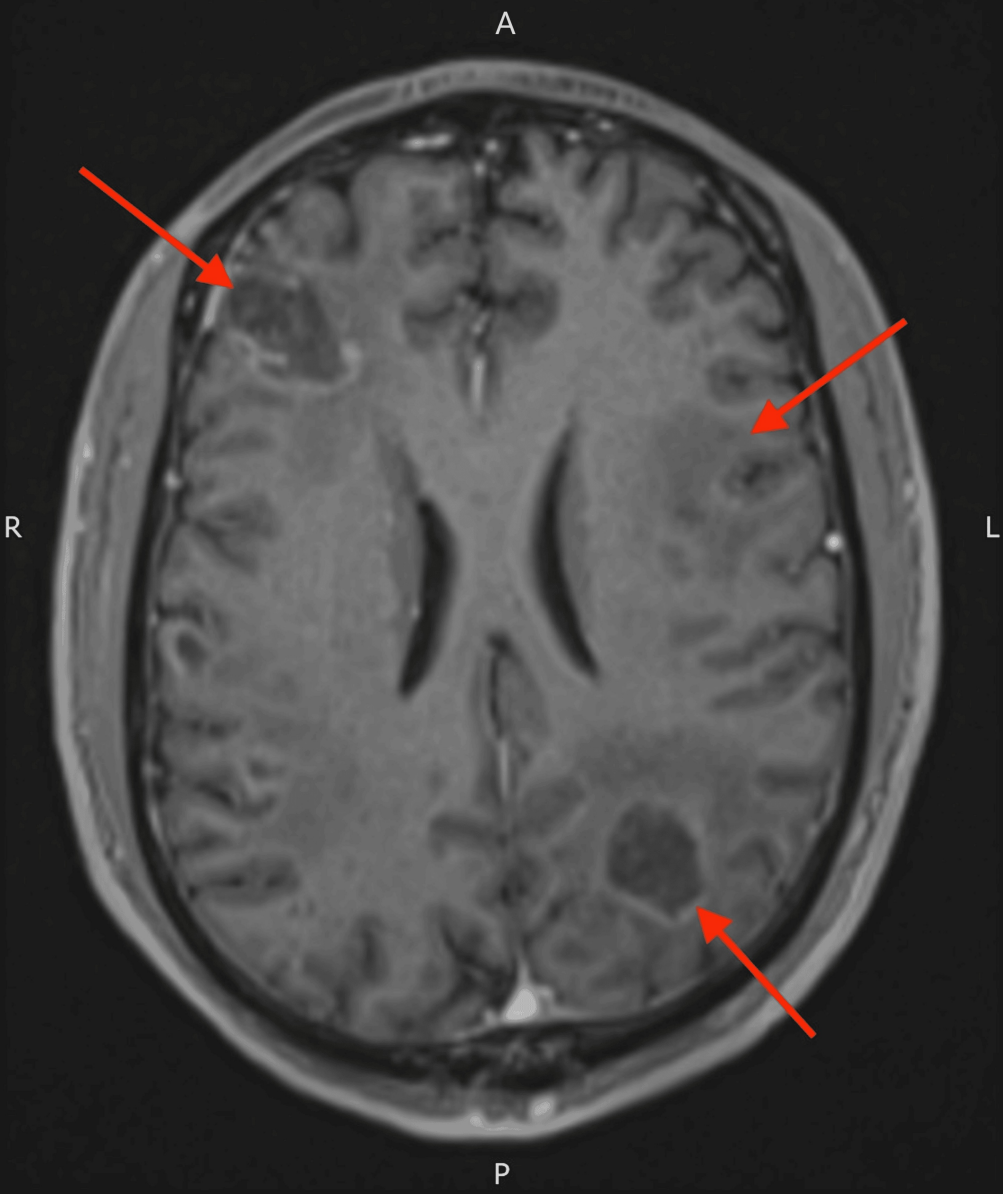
MRI of the head with contrast showing reduction in the size of the brain lesions and reduction in surrounding oedema MRI: magnetic resonance imaging
